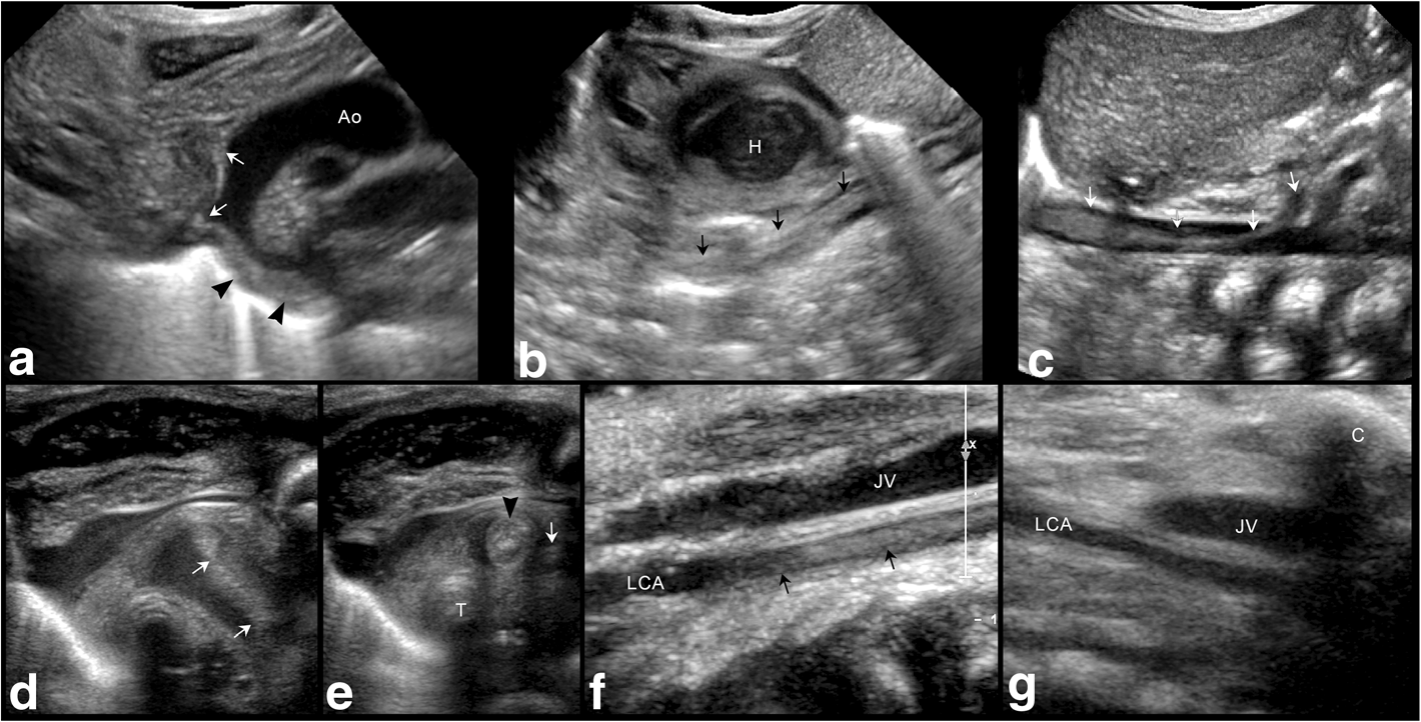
Vascular ultrasound. *Upper row* showing sagittal images of the embolus in the a aortic arch (*arrowheads*) with its extention into the innominate artery and the left carotid artery (*white arrows*); b descending aorta (*arrows*) and c abdominal aorta (*arrows*) with its end just above the celiac trunk. *Lower row* showing axial images of the upper mediastinum d with the embolus in the aortic arch (*white arrows*) and e the innominate artery (*arrowhead*) and in the left carotid artery (*arrow*); sagittal images of the neck f with the embolus within the supraclavicular part of the left carotid artery (*arrows*), g which is no longer visible 2 days later. Ao – aorta, H – heart, T – trachea, JV – jugular vein, LCA – left carotid artery, C - clavicula
